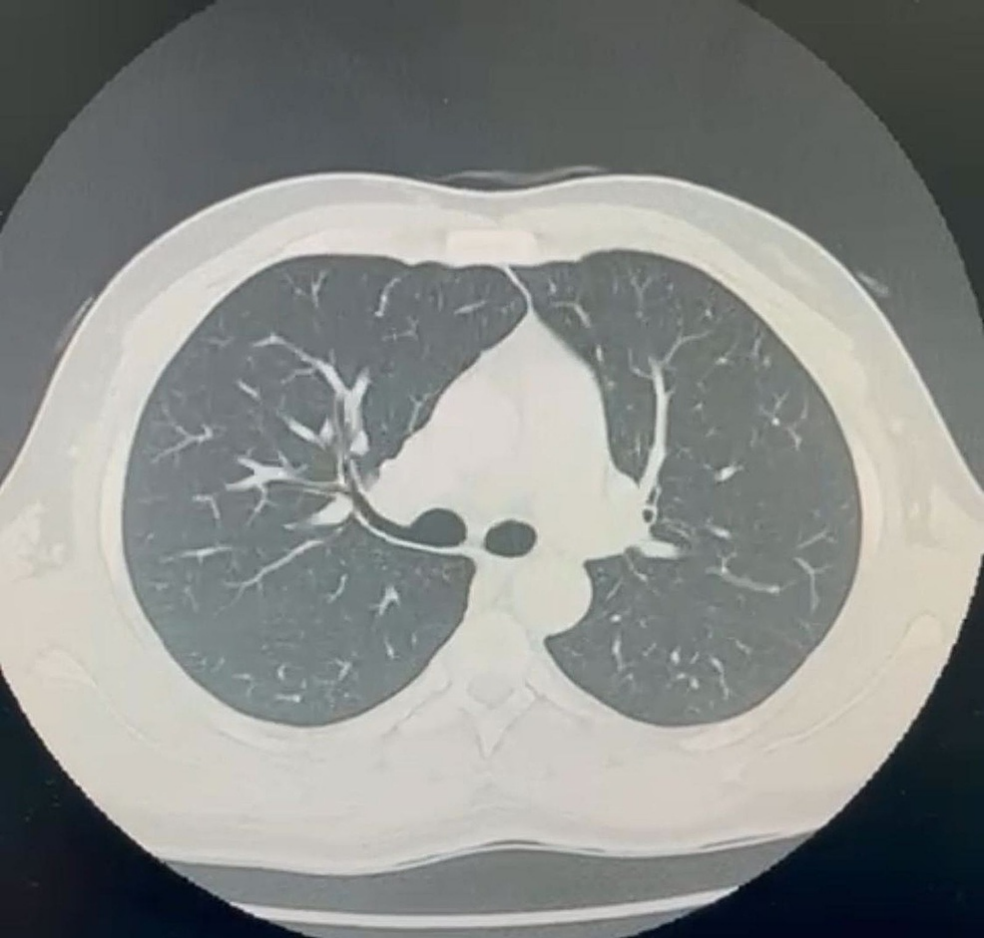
CT scan of the chest showing no metastasis to the thoracic cavity with overall normal appearance and vasculature.
